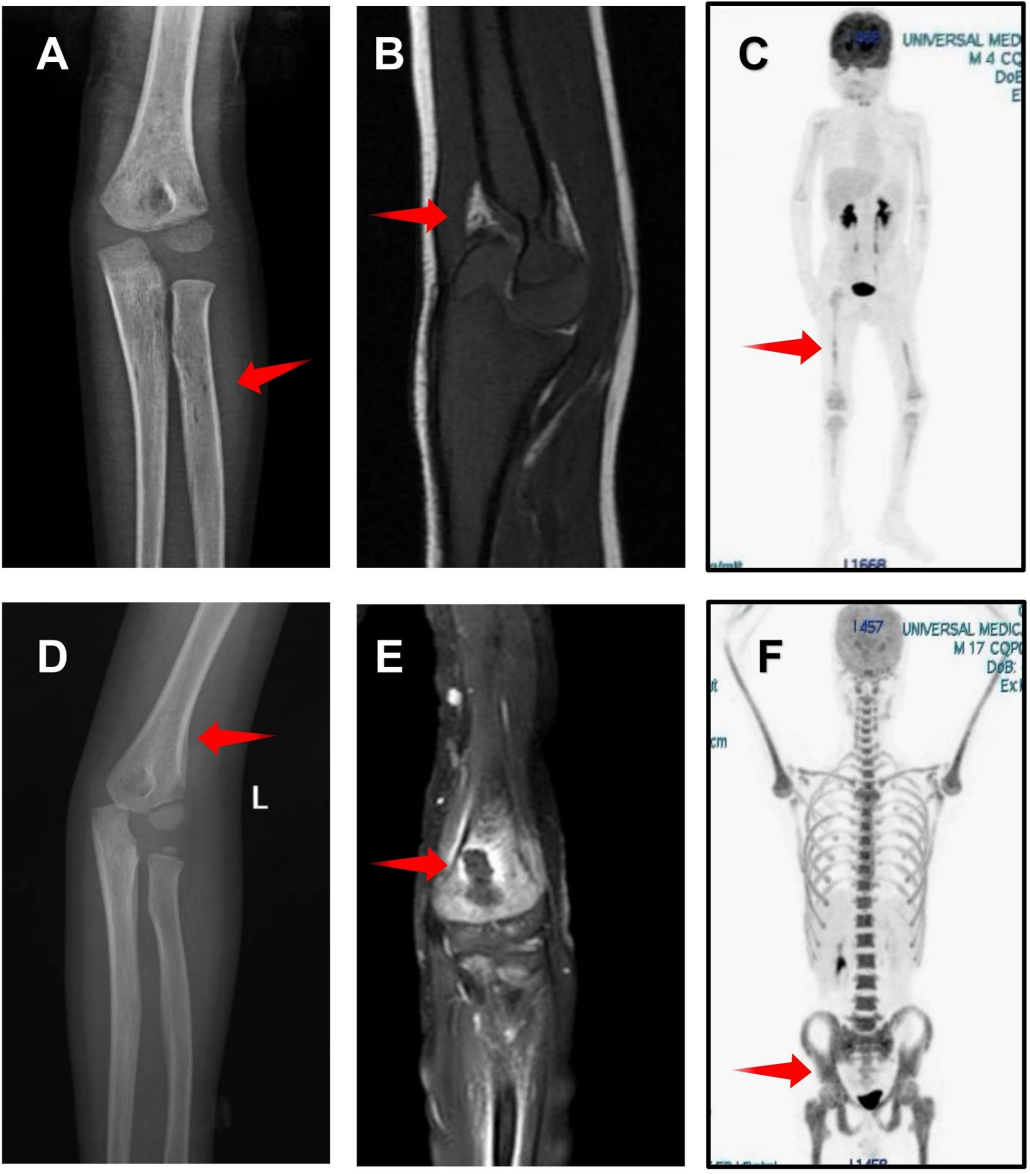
Imaging data of patients. (A) Anteroposterior radiograph of the left elbow joint of case 1. (B) T1 sagittal of the left elbow joint of case 1. (C) PET/CT of case 1 after the first session of chemotherapy. (D) Anteroposterior radiograph of the left elbow joint of case 3. (E) T1 sagittal of the left elbow joint of case 3. (F) PET/CT of case 2. PET/CT, positron emission tomography/computed tomography.
